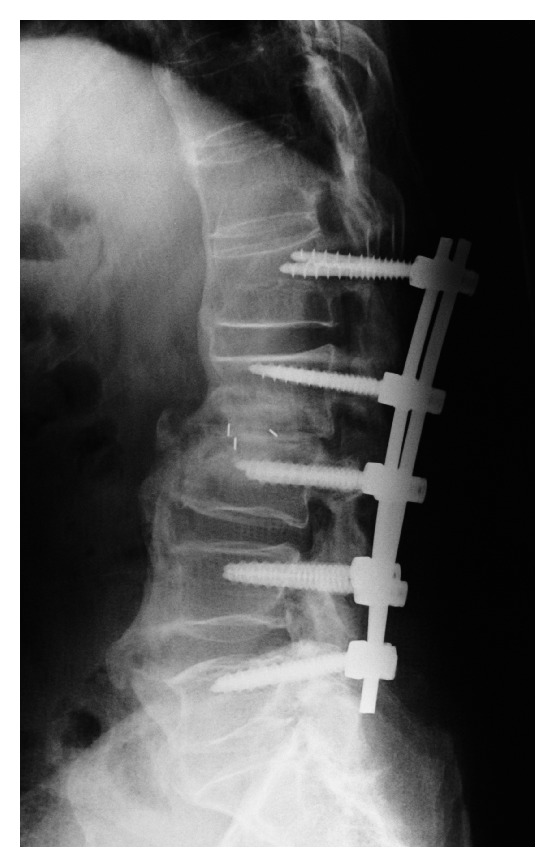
Lateral plain X-ray image obtained 10 weeks after the first surgery, showing loosening and back-out of the pedicle screw at L1-L2.
